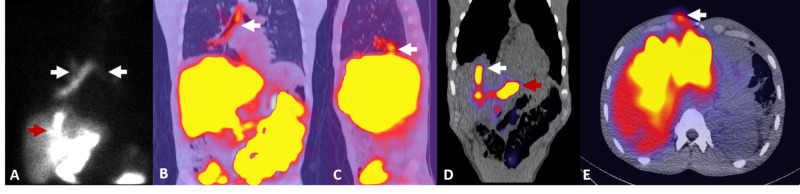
A 40-year-old male and victim of gun violence with surgical history of laparotomy, left hepatectomy, cholecystectomy and removal of two foreign bodies. (A) Anterior planar image of hepatobiliary scintigraphy shows abnormal activity which partially fills subdiaphragmatic fluid collection (red arrow) and abnormal activity in the bronchial tree, consistent with bronchobiliary fistula (white arrows). (B) Coronal SPECT/CT shows activity in the bronchial tree (white arrow). (C) Sagittal SPECT/CT reveals location of bronchobiliary fistula (white arrow). (D) Coronal SPECT/CT shows subhepatic biliary fluid collection (red arrow) and extention of biliary tract to hepatic dome (white arrow). (E) Axial SPECT/CT reveals biliarycutaneus fistula (white arrow).
